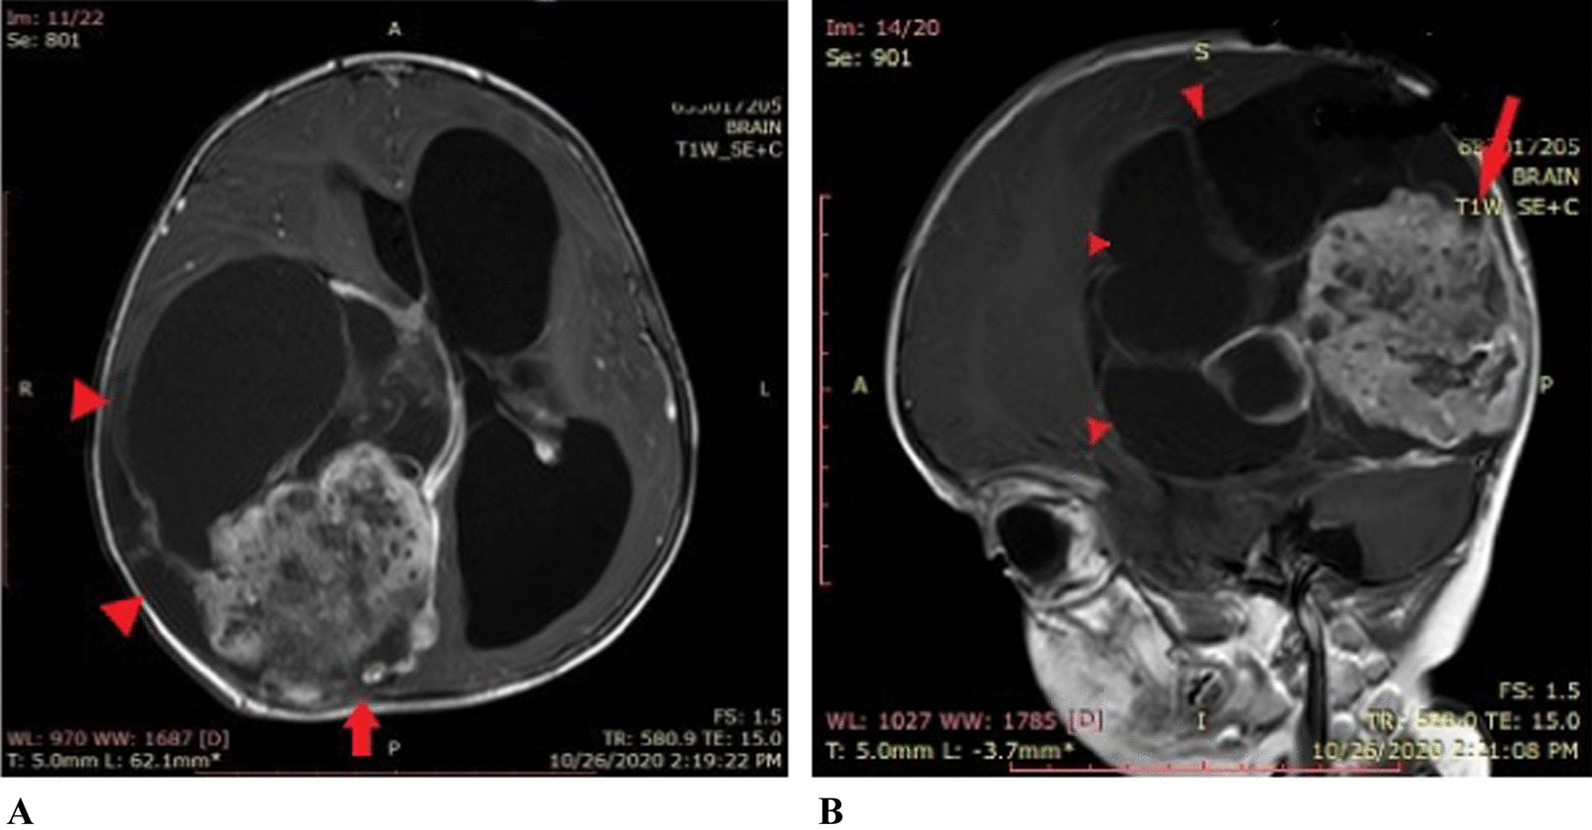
A, B Pre-operative MRI post-contrast: Axial and sagittal images show a heterogeneously enhancing predominantly cystic mass (red arrow heads) in the right parietal occipital location with a solid heterogeneously enhancing component attached to the dura (red arrow)
